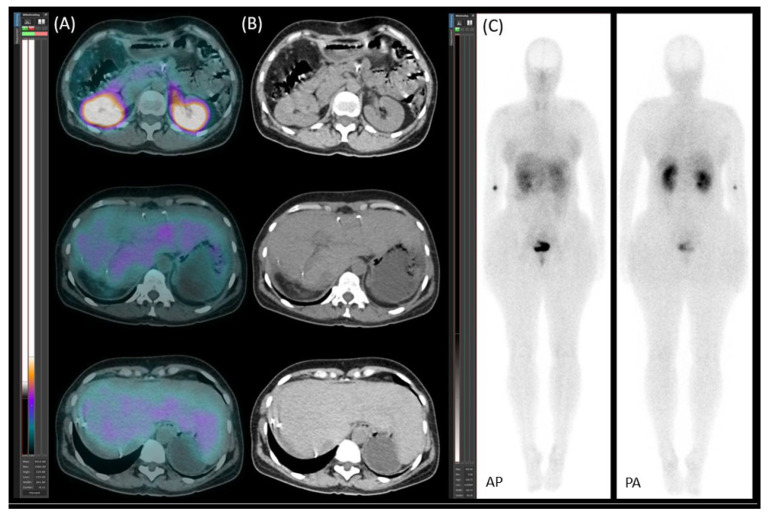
[^99m^Tc]Tc-EDDA/HYNIC-TOC scintigraphy with SPECT/CT. (A) Transaxial SPECT/CT, (B) transaxial CT, and (C) planar images (AP—anteroposterior; PA—posteroanterior) reveal no abnormal lesions.
